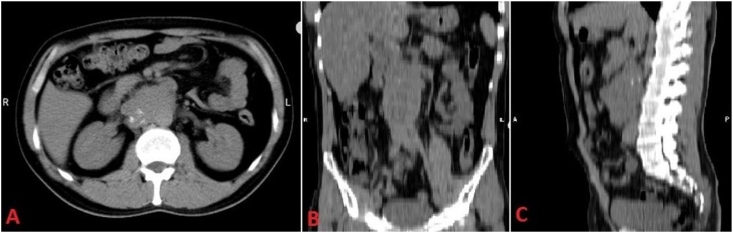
Retroperitoneal mass encasing the inferior vena cava and right renal vein with signs of calcification within the mass; Axial (A) Coronal (B) and Sagittal (C) sections of CT scan Imaging study.
